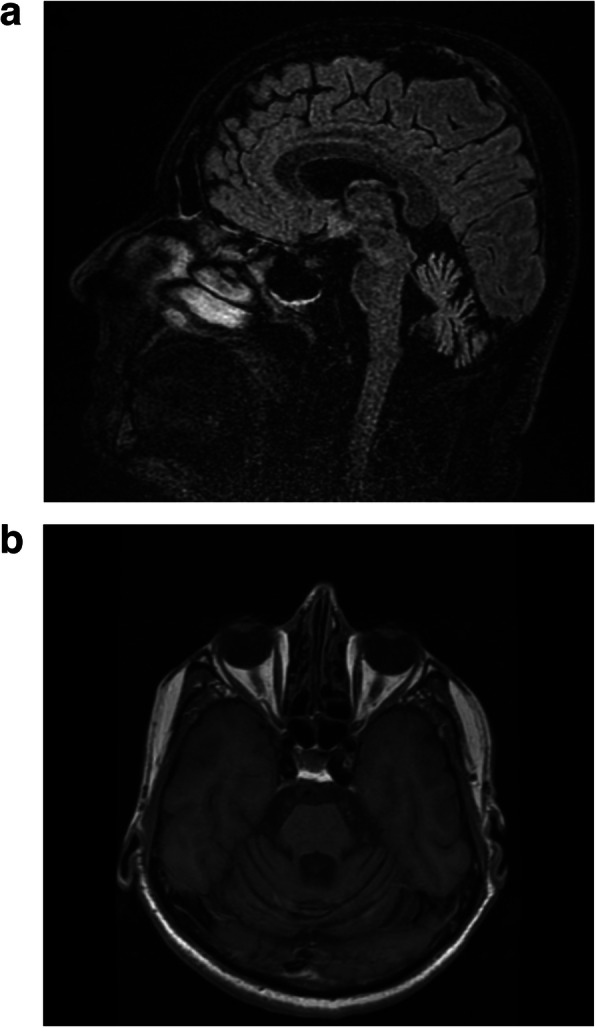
Patient brain MRI. Patient brain MRI performed at the age of 58 years, showing cerebellar atrophy and mild brainstem atrophy. a) Sagittal FLAIR image. b) Axial T1-weighted image
